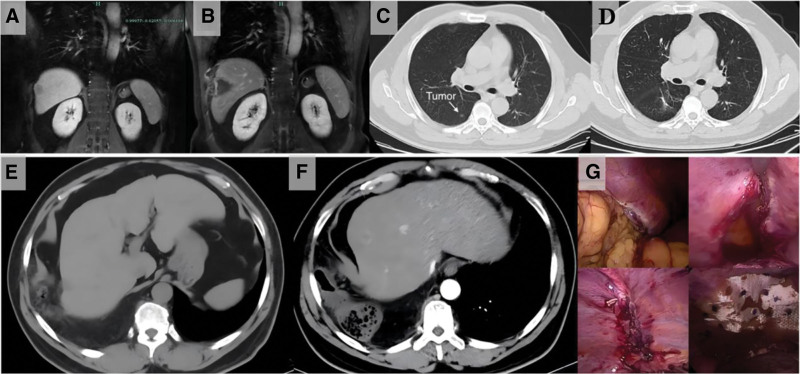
(A, B) MR images of hepatocellular carcinoma before and after RFA, revealed that the right diaphragm was injured. (C, D) CT images of the pulmonary adenocarcinoma before and after thoracoscopic wedge resection, revealed that it was not the location of the IDH. (E, F) Nine months after RFA, CT revealed a IDH with omentum and a small part of colon though the diaphragm defeat by RFA. The next day, the patient experienced right upper abdominal pain. Enhanced abdominal CT revealed a significant progress of the IDH. (G) Emergency laparoscopic surgery was performed to repair this IDH. CT = computerized tomography, IDH = iatrogenic diaphragmatic hernia, RFA = radiofrequency ablation.
